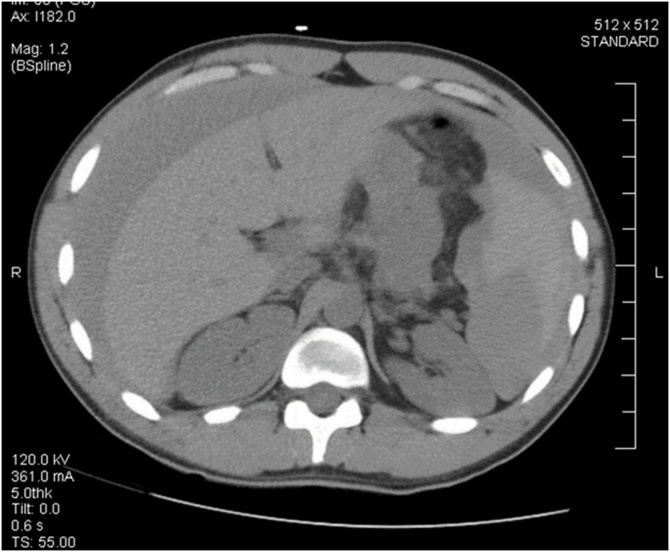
Axial view of CT scan of abdomen.
